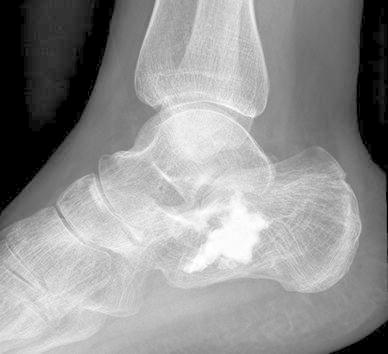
Four years postoperative lateral radiograph showing reconstruction and restoration of the thalamic surface
